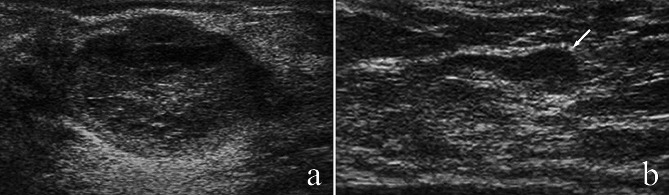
Ultrasonography of a PMBC tumor with ALNM. (a) Ultrasonogram shows a heterogeneous tumor with irregular shape, circumscribed margin and posterior enhancement. (b) Metastatic lymph nodes in the armpit with heterogeneous thickening of cortex (white arrow)
